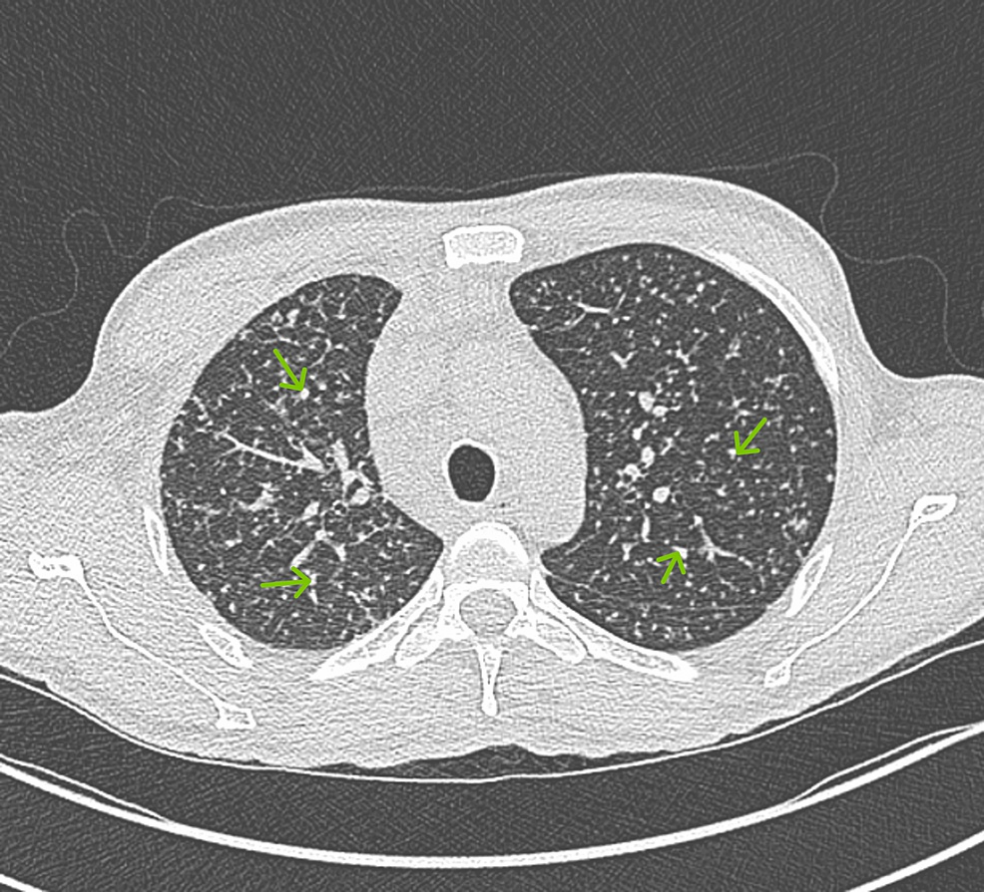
A CT scan of the lung A miliary pattern of micronodular intra-alveolar infiltrates is seen throughout the imaged portions of the lungs (green arrows). Retroperitoneal and mesenteric lymphadenopathy was found with multiple mildly enlarged lymph nodes.
